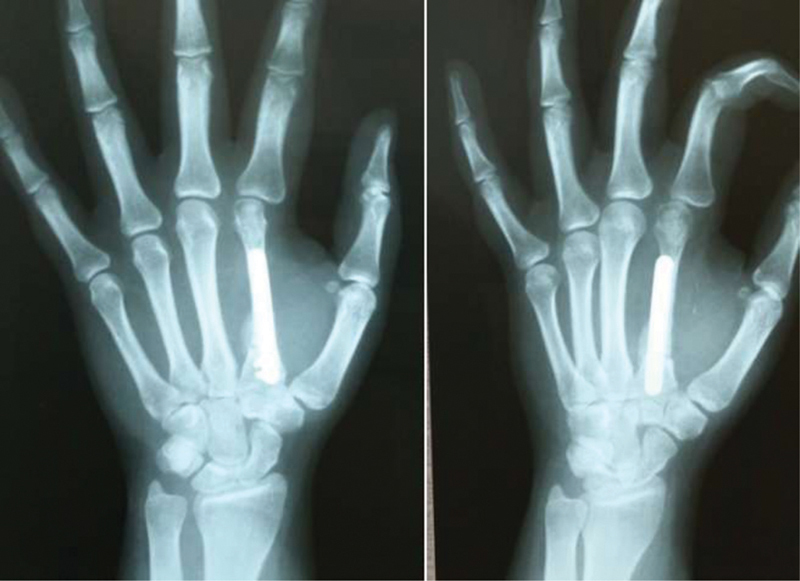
Patient in case 1 with 8.5 months after tumor resection and reconstruction with free osteoarticular metatarsus autograft. Radiograph 75 days after the iliac graft, showing complete consolidation and integration.
